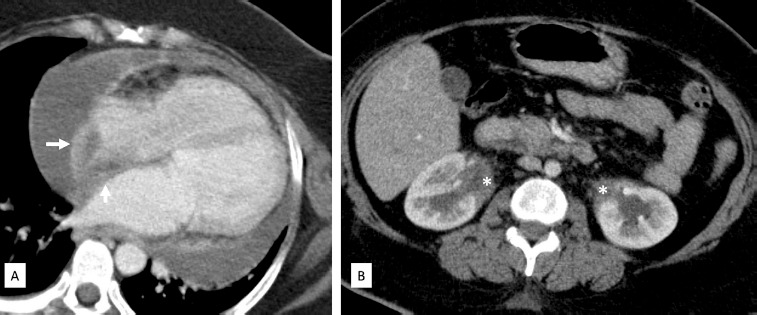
MRI brain T1 pre-contrast (A) and post-contrast (B) images in sagittal (A) and axial (B) views show a brightly enhancing lesion (the white block arrow) of a size of 2x2 cm in pineal gland which was causing aqueductal compression
